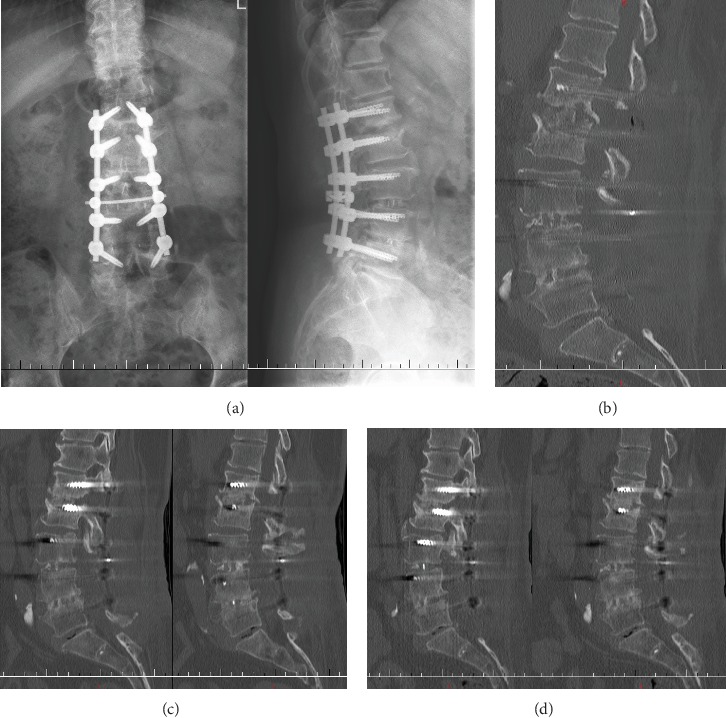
Anterior-posterior and lateral lumbar radiographs 1 week after revision. The upper instrumented level was extended to L1 (a). Sagittal lumbar CT 1 week after revision. The infective tissues at the L1-2 space were debrided and then an iliac crest autograft was implanted (b). Sagittal lumbar CT 6 weeks after revision. The surgical space tended to fuse with well-placed instruments (c). Sagittal lumbar CT 6 months after revision showed satisfactory fusion sign in the infective space (d).
